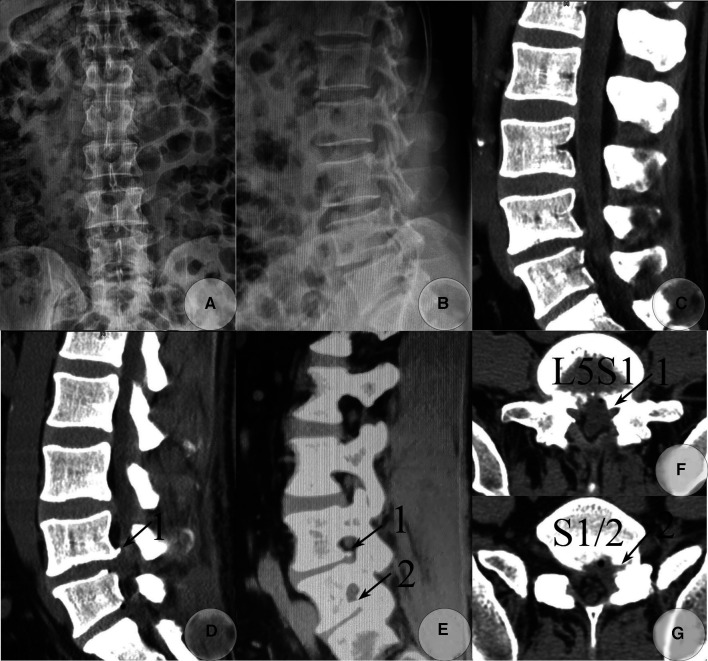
Postoperative lumbar radiograph and CT images taken on 26 and 27 December 2019, respectively. AP (A) and lateral view (B) of the lumbar radiograph. Postoperative CT images (C–G) detected the majority of calcified fragments that were removed. The calcified base at the posterolateral corner of the L5 inferior endplate was left asymptomatic (soft tissue window), and the calcified shell of the herniated S1-2 disc and osteophytes from the inferior endplate of S1 within the S1-2 foramen was also not removed. (C) Sagittal reconstructed CT image through the left paracentral plane. (D) Sagittal reconstructed CT image through the left recess. (E) Sagittal reconstructed CT image through the left foramen plane. (F) Raw transverse CT image through L5S1 level. (G) Raw transverse CT image through S1-2 level. (Arrow 1) Residual osteophytes at the posterolateral corner of the L5 inferior endplate. (Arrow 2) A residual calcified shell of the herniated S1-2 disc and osteophytes from the inferior endplate of S1 within the intervertebral foramen.
